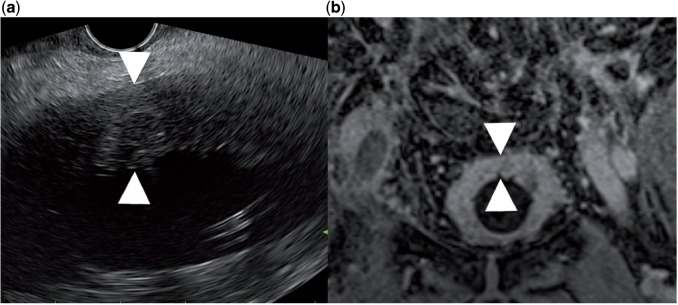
(a) Transrectal endoscopic ultrasound examination revealed an 11 mm thickening of the urinary bladder. The endoscope is orientated towards the anterior wall of the rectum. (b) This finding was confirmed by means of magnetic resonance imaging. Fine needle aspiration cytology disclosed a linitis-plastica carcinoma of the urinary bladder.
